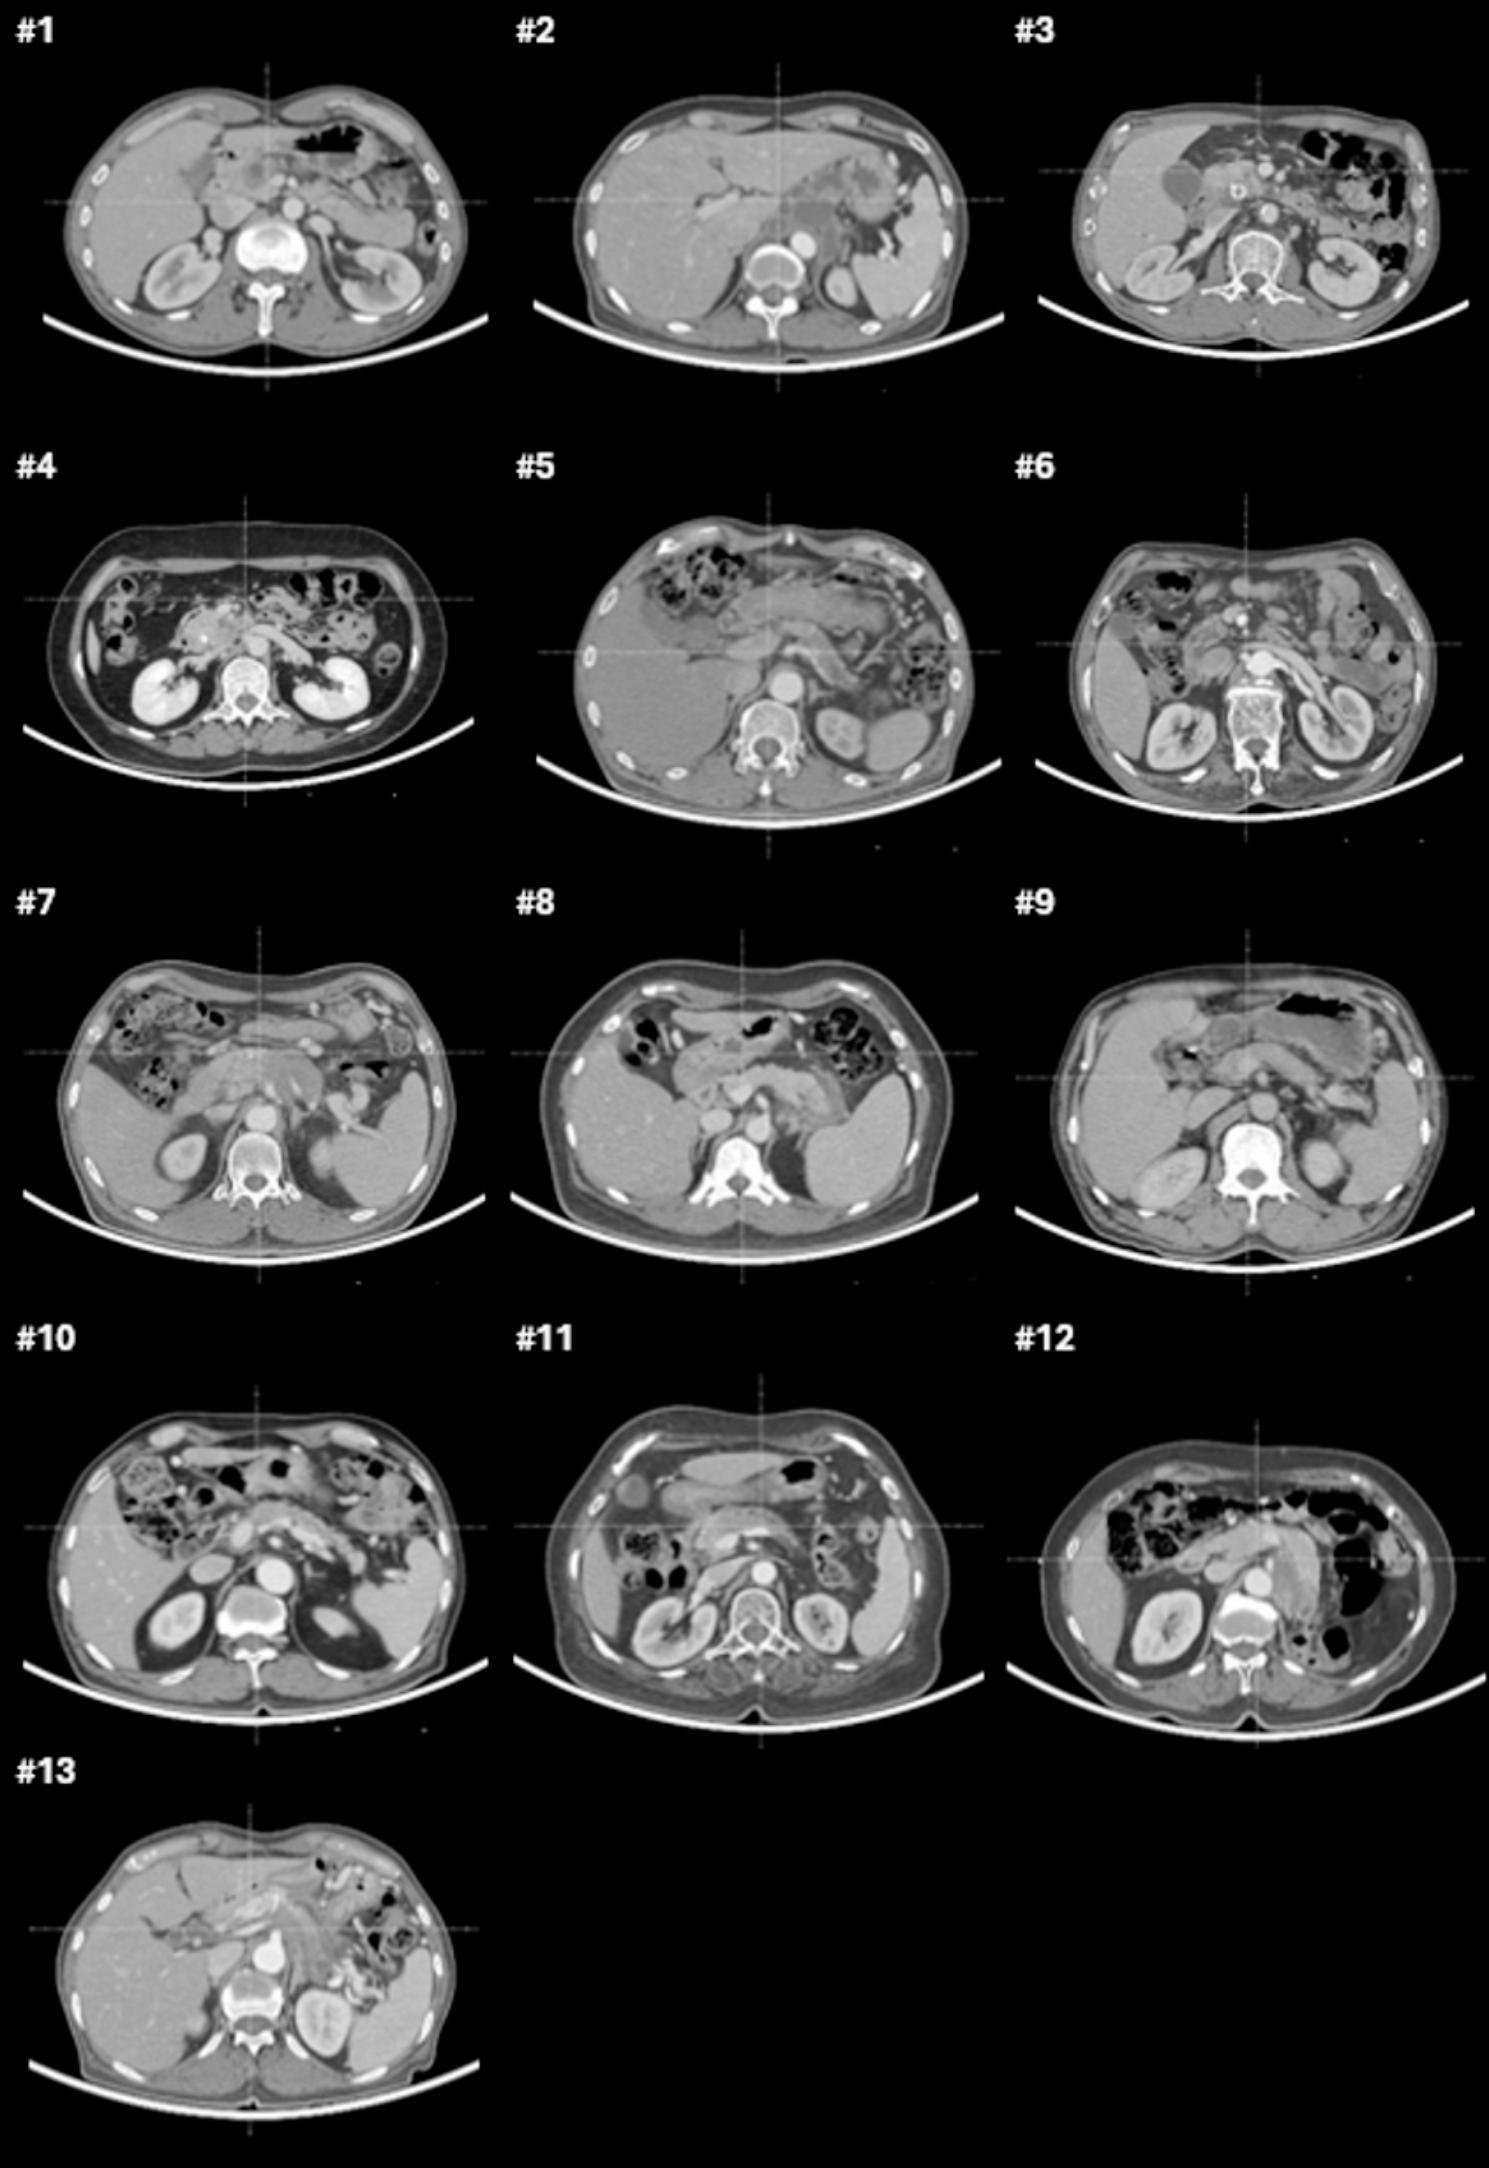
Axial CT slice images taken at the reference point are provided for all 13 patients, offering a visual representation of the anatomical structures and electrode placements used in the study.
